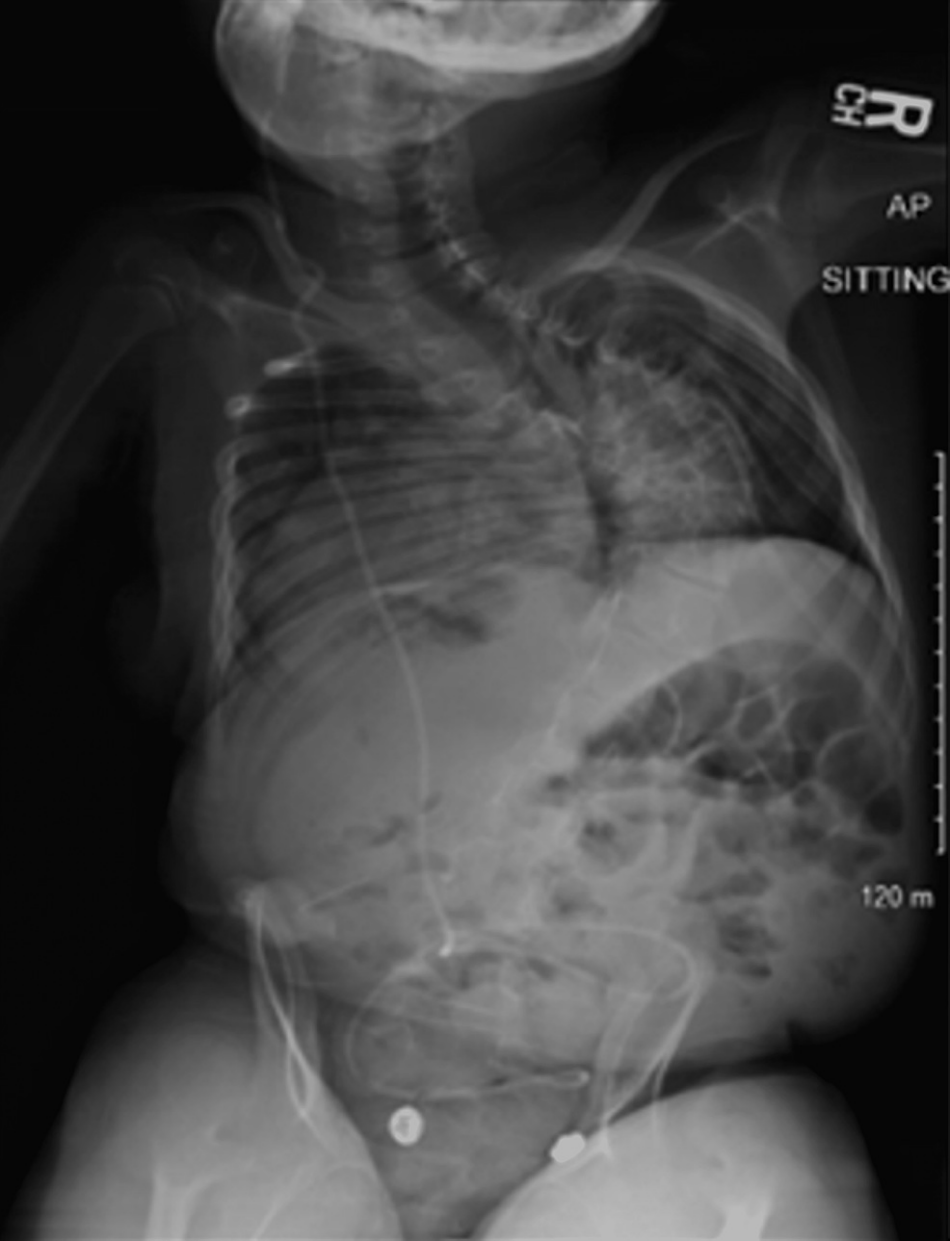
Posterior-anterior plain radiograph performed before index surgery for MAGEC rod placements at eight years of age.
